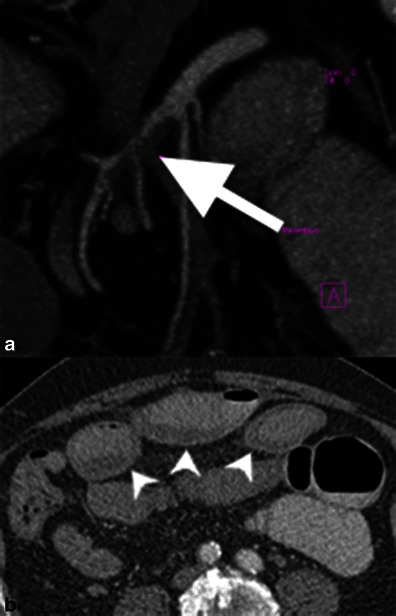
SMA thrombosis. a Contrast-enhanced, coronal reformatted CT images demonstrate an eccentric filling defect in the proximal SMA, corresponding to thrombus formation. b Axial contrast-enhanced CT image shows oedematous bowel wall thickening of small bowel loops (*white arrowheads*) and mesenteric congestion. This patient subsequently improved with conservative anticoagulation therapy
